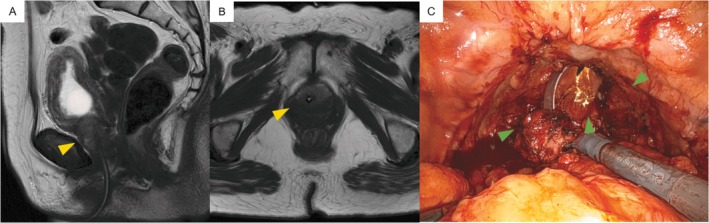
Sagittal (A) and axial (B) T2‐weighted magnetic resonance images of Case 1 before chemotherapy. The yellow arrows indicate a 23‐mm mass in the urethral diverticulum. Tumor invasion into the anterior vaginal wall was suggested. (C) The green arrows indicate that the anterior vaginal wall, external urethral meatus, and levator ani muscle within the endopelvic fascia were resected en bloc.
